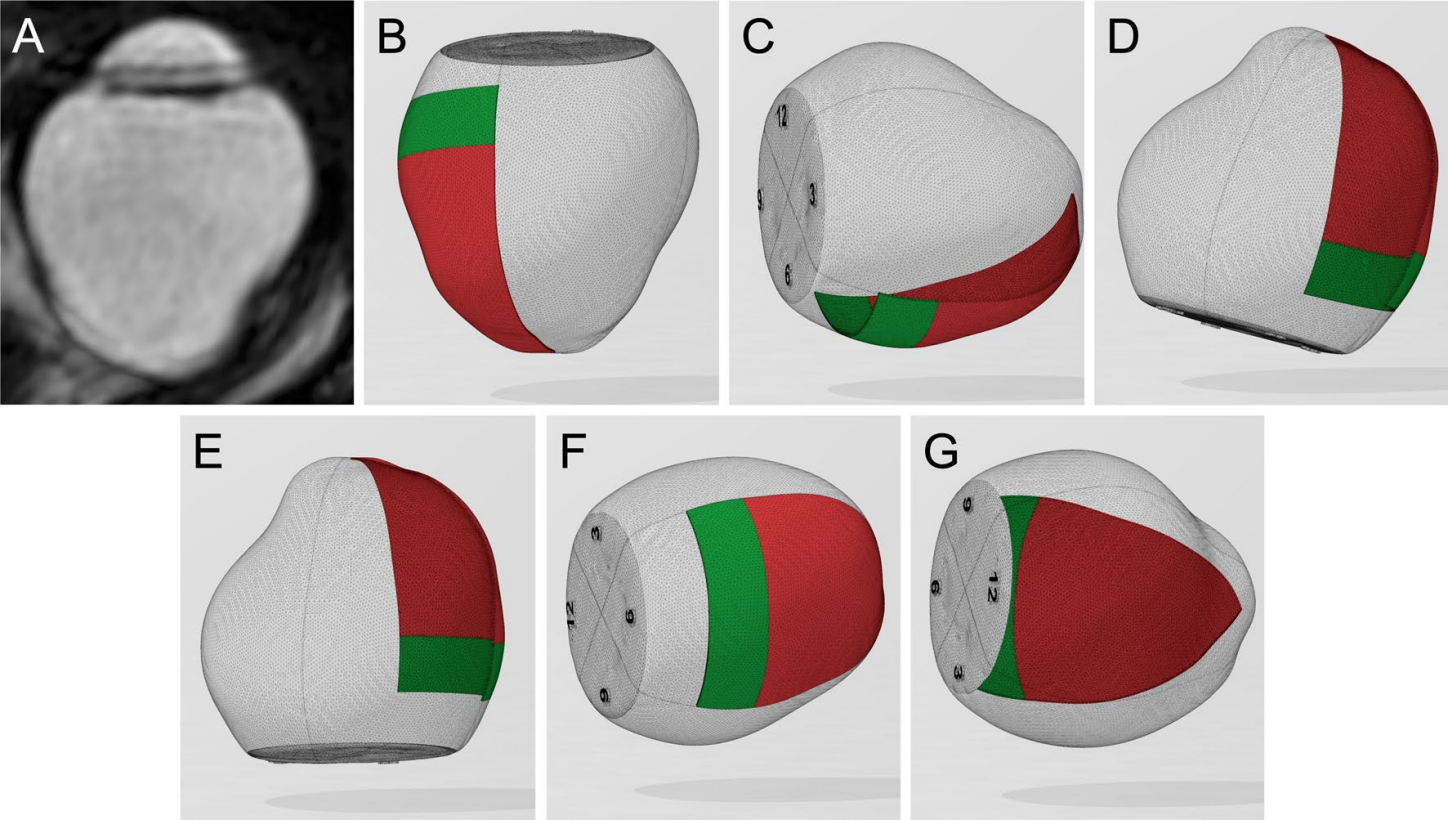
Directions of the three-dimensional vitreous cavity in case 1. (A) The real MRI image, (B) Supine, (C) sitting, (D) prone with closed eyes, (E) prone, (F) lower temporal, and (G) lower nasal positions. The inferior-anterior and inferior-posterior retina are colored green and red.
